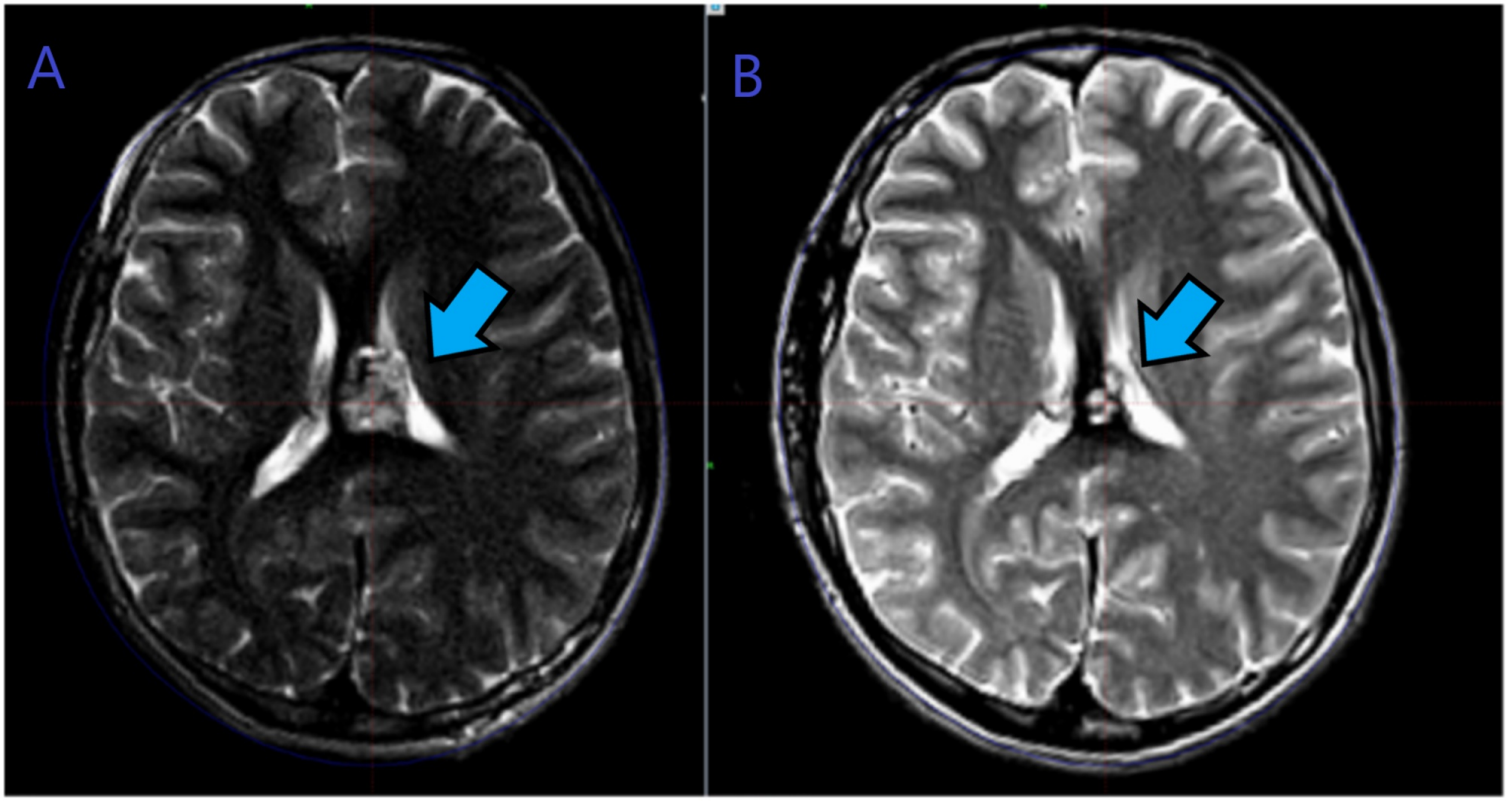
A. MRI brain T2 sequence showing the “pop-corn appearance” of cavernous angioma. Left medial parietal area involving the corpus callosum at the time of Gamma Knife radiosurgery treatment. B. MRI axial cut T2 sequence showing more than 50% regression in the lesion size, six years after the Gamma Knife treatment. MRI: magnetic resonance imaging
